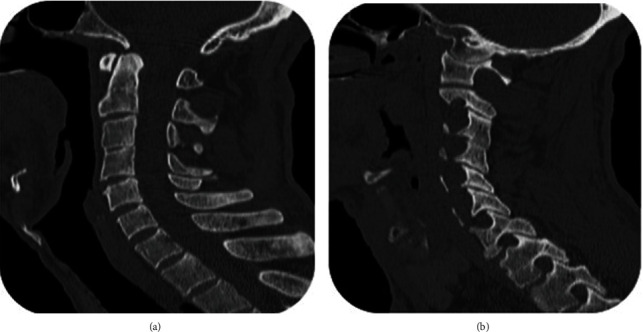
Sagittal spiral CT scan of cervical spine, showing C3-C4 partial fusion (a) and expanded intervertebral foramina mostly at C1-C2 level (b).
